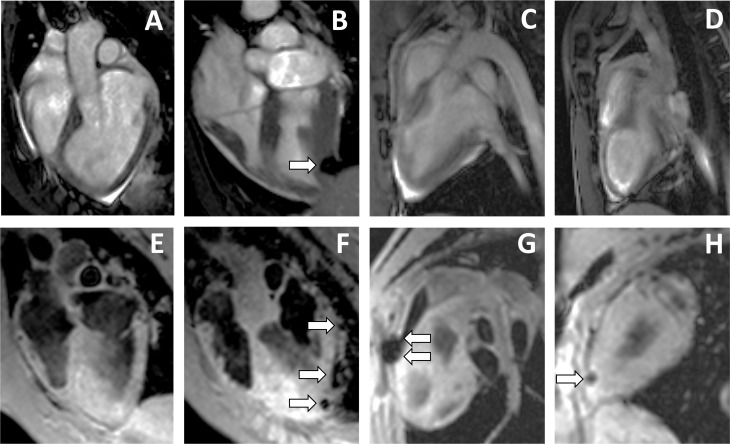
In vivo* cell tracking of intrapericardially delivered pBM-MSCs by cardiac MRI in infarcted heart. A total of 100x10^6^ SPIO-labeled pBM-MSCs cells were injected into the pericardial cavity. The MRI was performed using 1.5T magnetic resonance technology. Images were acquired in four chambers view (A-D) and using a T2-star gradient echo image (E-H). Images taken at day 3 post injection (B-D, F-H) are represented together with the corresponding control images (A, E) taken before injection and one week after the infarction induction. The arrows indicate the location of SPIO-labeled cells.
